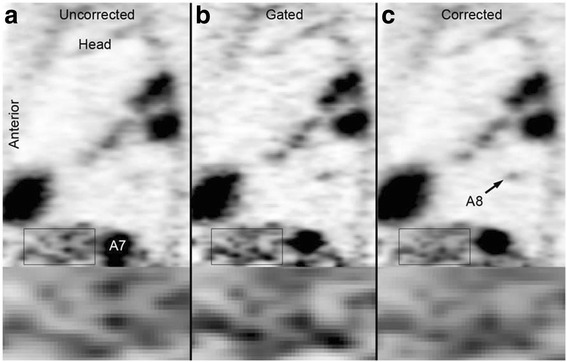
Comparison of uncorrected (a), gated (b), and corrected (c) sagittal PET image slice featuring lesions A7 and A8 in patient A. Multiple lesions and inflammatory areas with increased 18F-FDG uptake in lung show enhanced delineation (This research was originally published in *Würslin C, Schmidt H, Martirosian P, Brendle C, Boss A, Schwenzer NF,* et al., “Respiratory motion correction in oncologic PET using T1-weighted MR imaging on a simultaneous whole-body PET/MR system, (Würslin et al. 2013)” J. Nucl. Med. Soc Nuclear Med; 2013;54:464–71. © by the Society of Nuclear Medicine and Molecular Imaging, Inc.)
